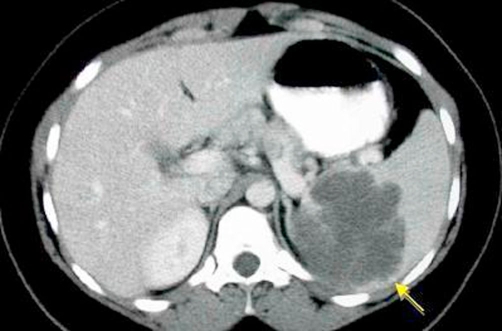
CT scan of the splenic cyst (arrow).
